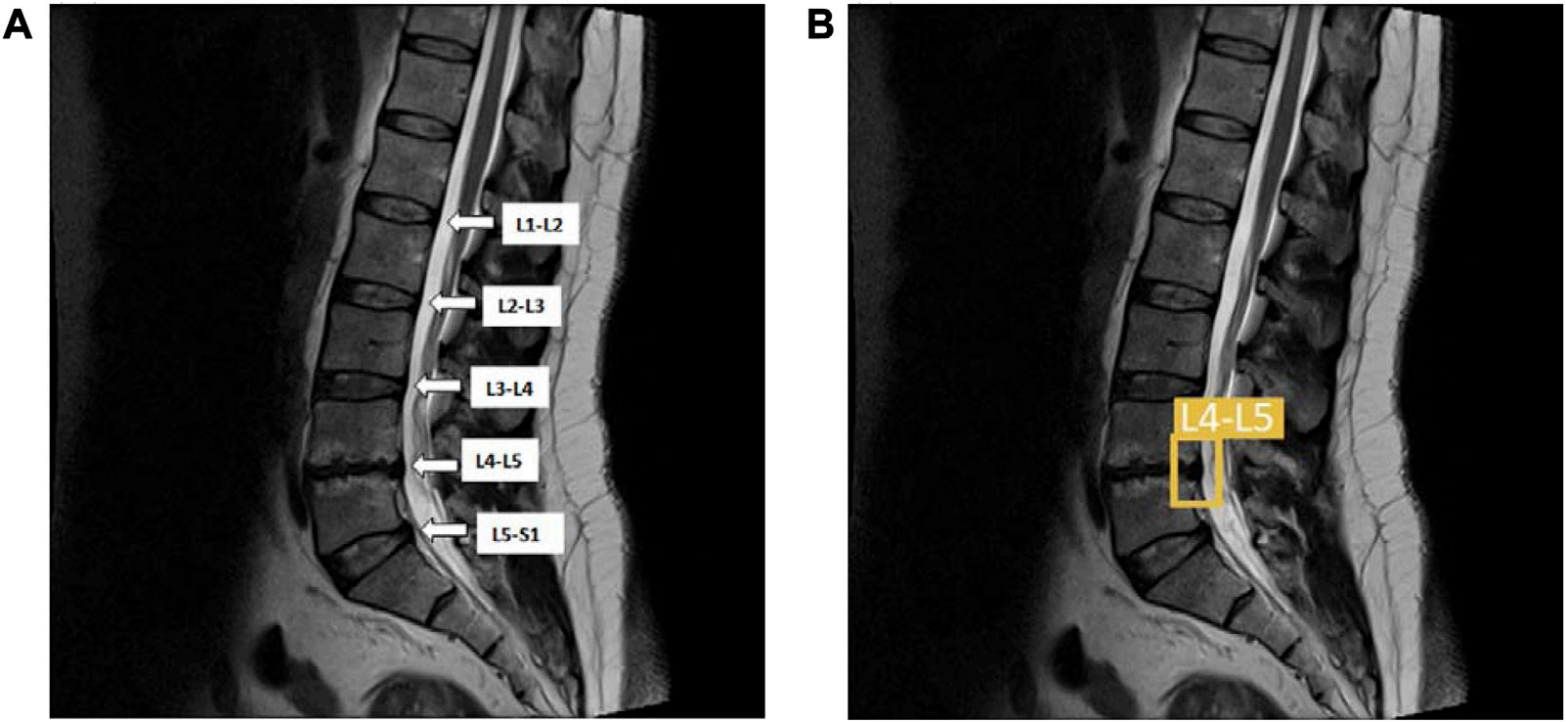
(A) The lumbar vertebrae and disc sections are from L1-L2, L2-L3, L3-L4, L4-L5, and L5-S1; (B) The same lumbar MRI displays LDH detection at L4-L5 and L5–S1. L1, first lumbar vertebra; L2, second lumbar vertebra; L3, third lumbar vertebra; L4, fourth lumbar vertebra; L5, fifth lumbar vertebra; S1, first sacral vertebra; LDH, lumbar disc herniation.
